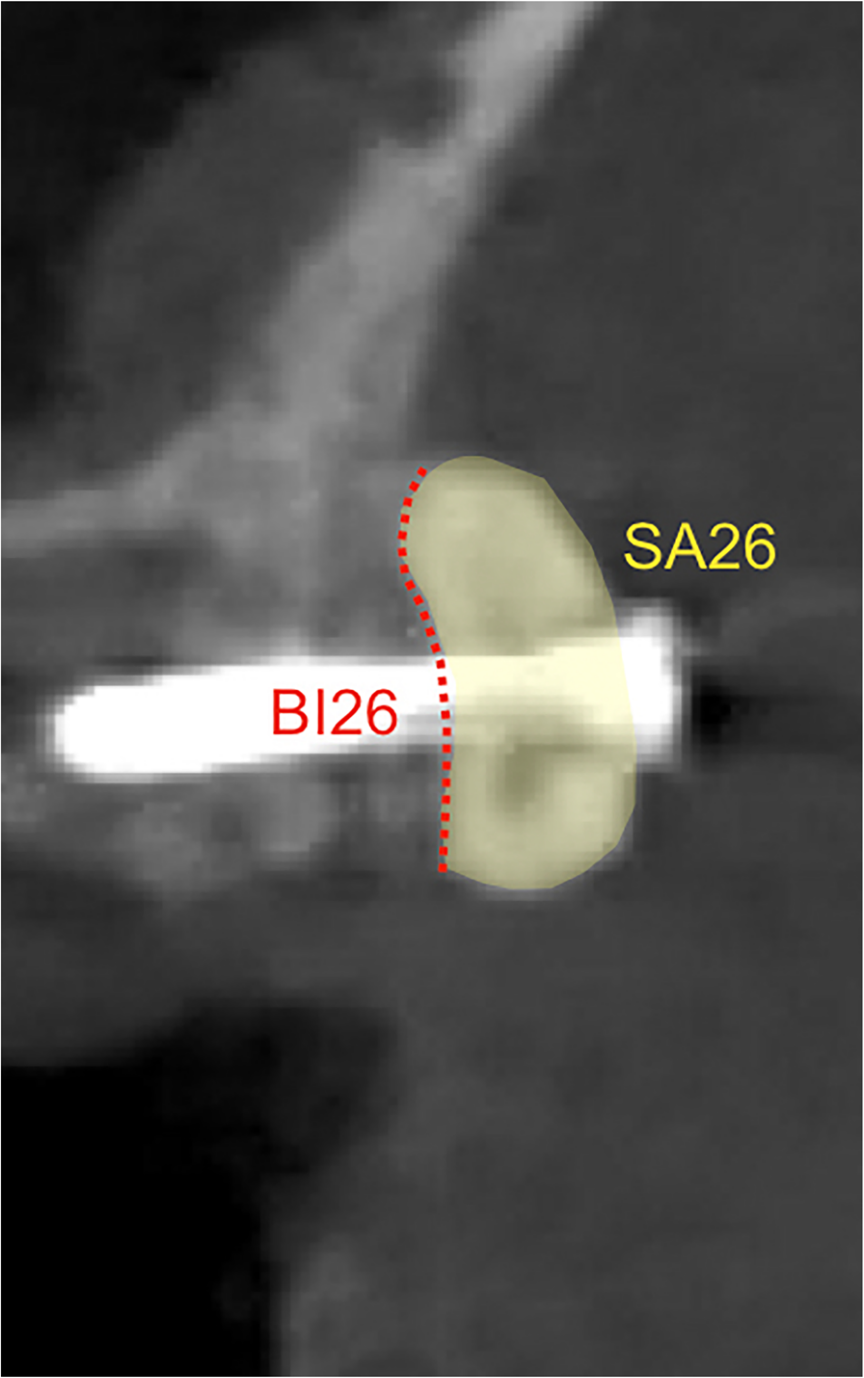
Radiographic assessments. Images of the coronal planes representing the most central aspect of the respective defect sites were analyzed for the basal graft integration (i.e., contact between the graft and the host bone in %) (BI26) and the cross-sectional grafted area (mm^2^) (SA26)
